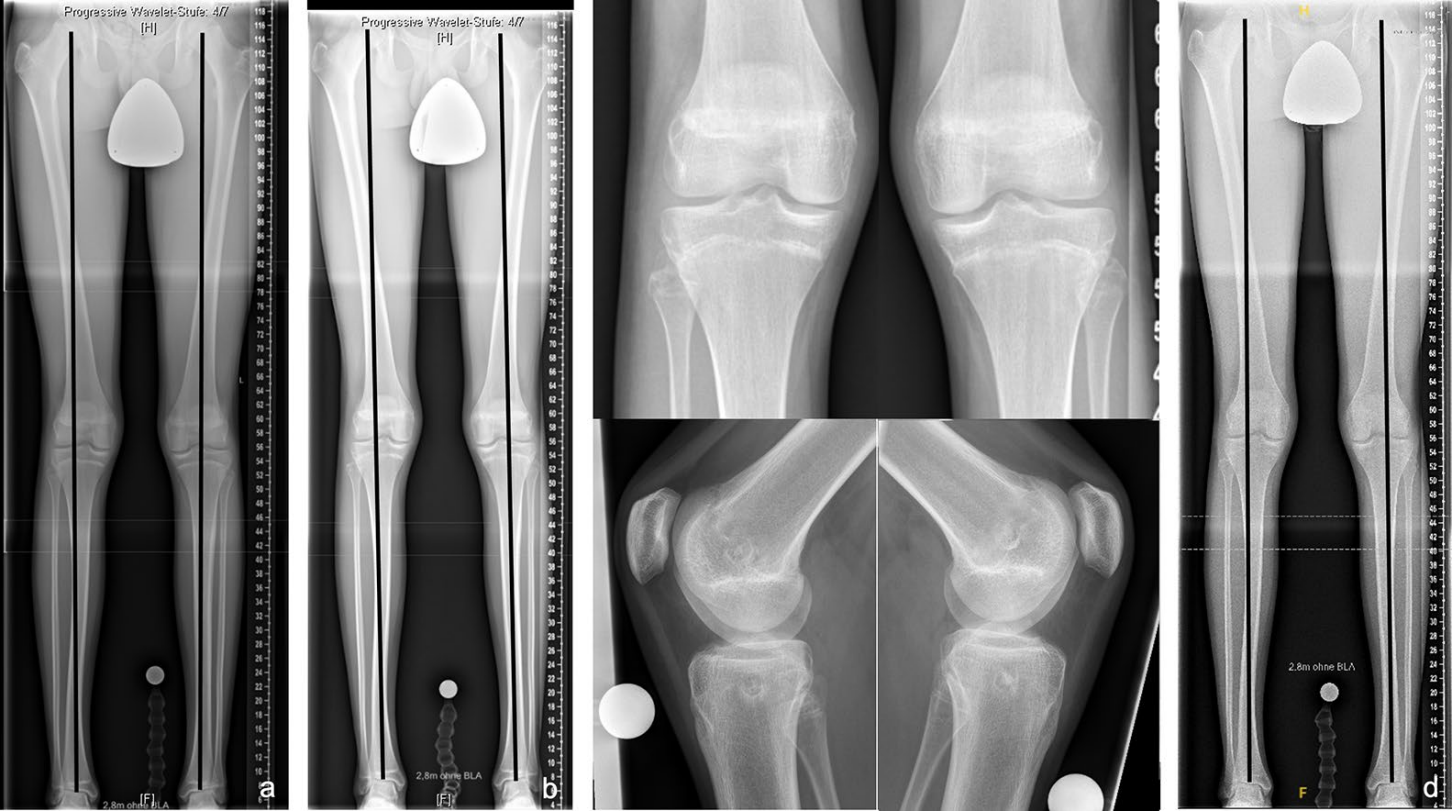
Results of bilateral permanent epiphysiodesis using a bone trephine (EpiStop™) around the knee in a girl with idiopathic (familial) tall stature. Anteroposterior long standing radiographs right before surgery at the age of 13 years (a), 6 months after surgery (b, c), and at skeletal maturity (d), showing physiological coronal alignment and equal leg lengths during and after treatment
